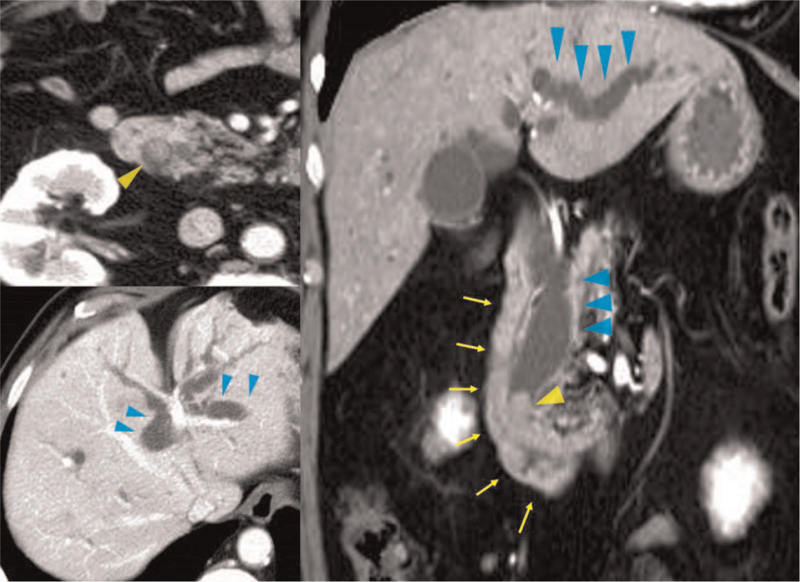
Contrast-enhanced abdominal CT revealed a 1 cm-sized tumor (yellow arrowhead) in the distal bile duct. The common bile duct and intrahepatic bile duct were dilated (blue arrowhead) due to this tumor. Wall thickening was seen in the second portion of the duodenum (yellow arrow). The endoscopic finding suggested the wall thickening as the mucosal lesion.
